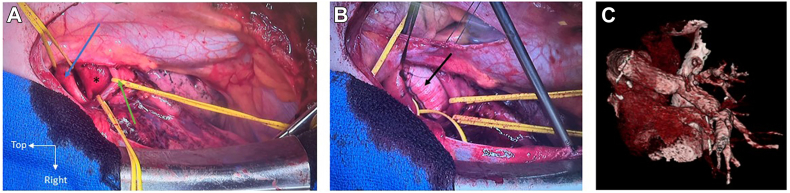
Intraoperative and postoperative views. (A) Intraoperative view showing the truncus anterior (blue arrow), the right superior pulmonary vein (green arrow), and the pulmonary artery trunk (star). (B) Intraoperative view showing the graft after completion of both anastomoses (arrow). (C) Posterior view of the computed tomography scan performed 3 months after surgery, confirming graft patency.
